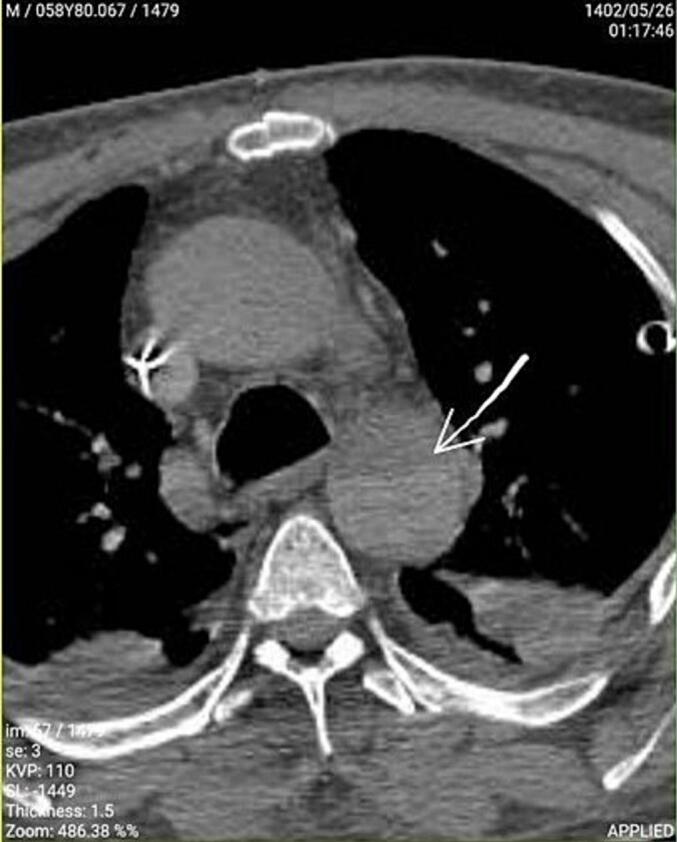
This CT angiography image shows the dissected section, which has been marked by a white arrow.
